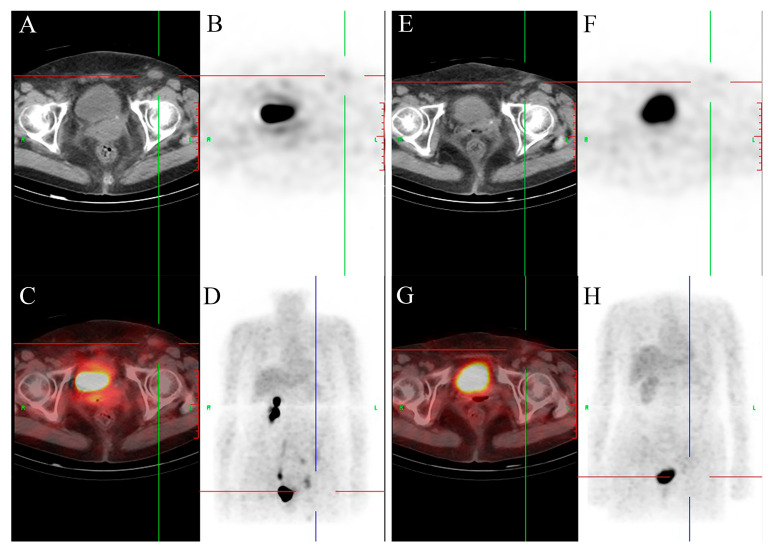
PET/CT evaluation of the left inguinal lymph node. Left panels (pre-treatment): (A) Axial CT image shows an enlarged left inguinal lymph node. (B) Corresponding PET image shows focal abnormal radiotracer uptake, indicating high metabolic activity. (C) Fused image confirms the hypermetabolic node corresponds to the anatomical enlargement. (D) Whole-body MIP shows a prominent hypermetabolic focus in the left inguinal region. Right panels (post-treatment): (E) Axial CT shows significant reduction in lymph node size. (F) PET and (H) MIP images show markedly decreased metabolic activity. (G) Fused image confirms lymph node shrinkage and metabolic reduction.
